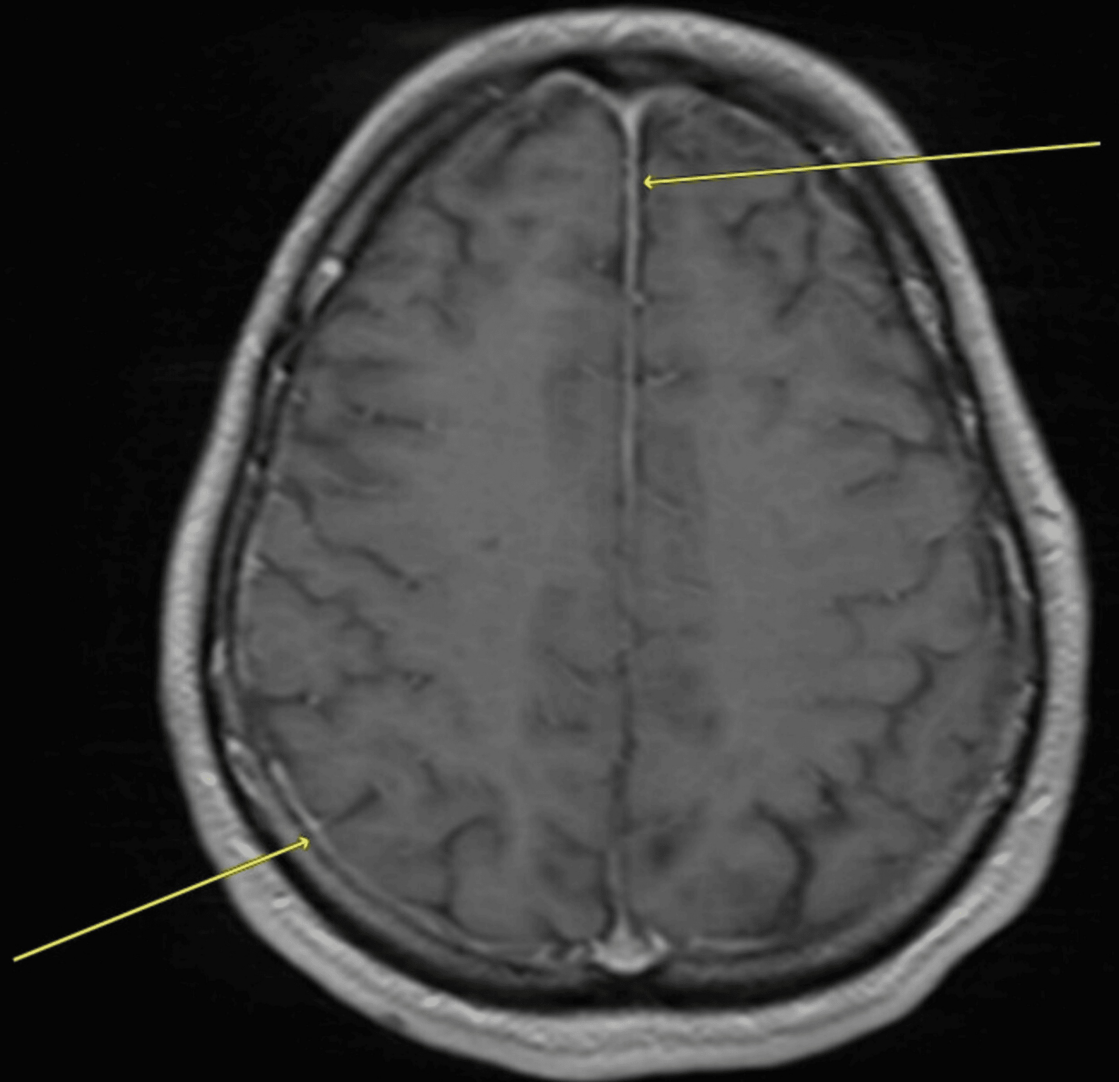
MRI scan’s T1 axial view with contrast showing leptomeningeal enhancement (arrows).
